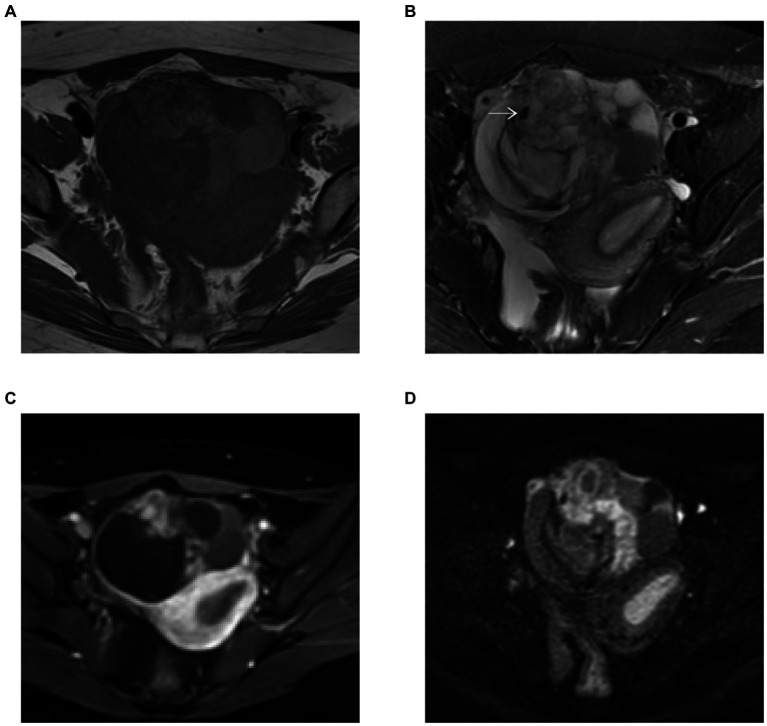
(A–D) Pelvis MR images. (A) T1-weighted image. MRI showed small foci of markedly low intensity (white arrow) on T2-weighted images (B) and contrast enhanced T1-weighted images demonstrated hyperenhancement within the solid component of the mass (C). This area corresponded to hyperintensity on DWI (b = 800) (D).
